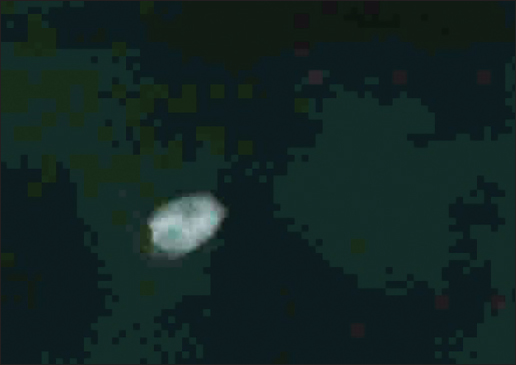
Radiograph of the lip showing the radiopaque tooth fragment.
